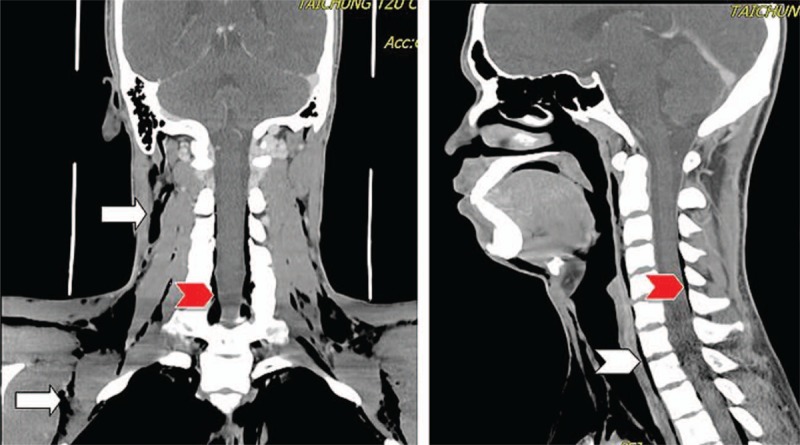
Contrast neck and thoracic CT demonstrating pneumomediastinum with extensive emphysema in the neck and chest wall (arrows). Free air was present in the paraspinal region (white arrowhead) and epidural space of the spinal canal (red arrowheads), which is termed as “pneumorrhachis.” CT = computerized tomography.
